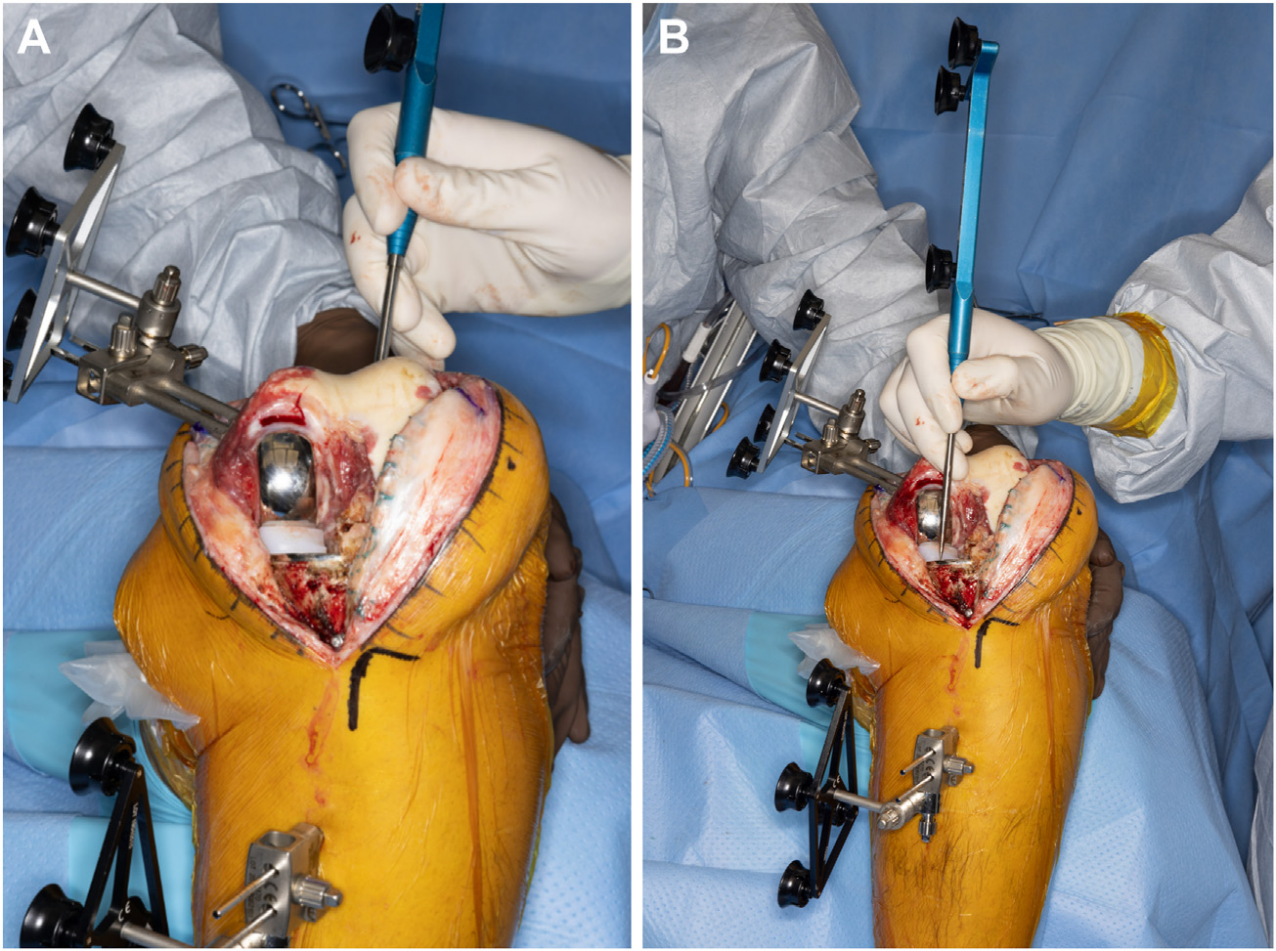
Bone registration. A sharp probe is used following on-screen instructions to register 40 individual osseous points along the distal femur (a) and proximal tibia (b). Note that the probe was directed along the metal tray and not the polyethylene liner of the tibial component. This permits robotic registration of the 3-dimensional anatomy previously ascertained by computed tomography scan preoperatively.
